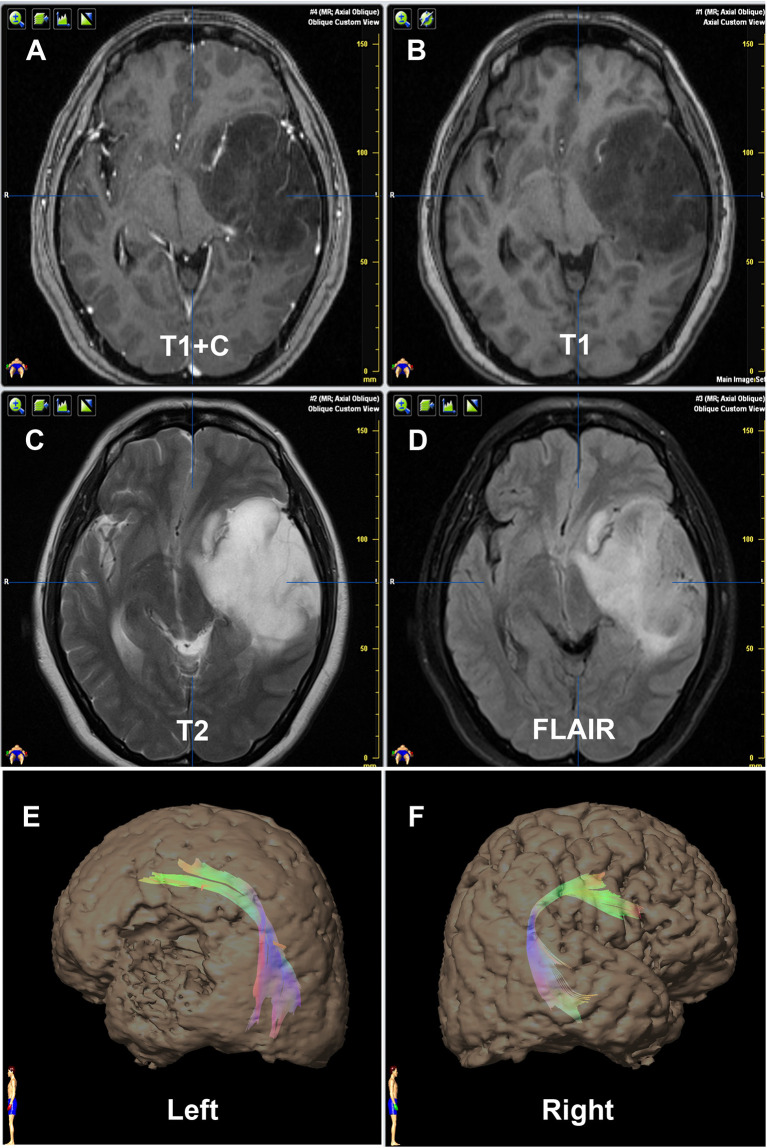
Contrast of bilateral AF by DTT of Patient No. 21. Panels (A–D) are preoperative MRI T1+C, T1, T2, and FLAIR, respectively, on one same transverse slice of Patient No. 21, who was diagnosed as primary LGG involving Broca’s area. Panels (E, F) show that the relative more fiber number and volume of AF are on the left hemisphere, which are in concordance with the language dominant side of Wada test.
